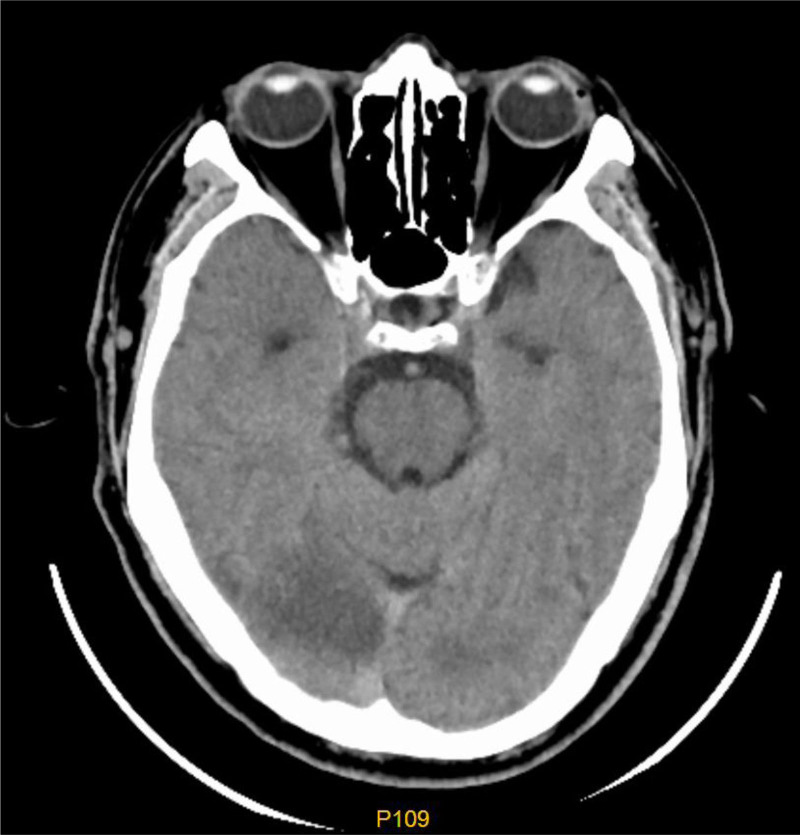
Plain CT scan of the brain (May 26, 2023) suggests cerebral infarction in the right occipital lobe. CT = computed tomography.
